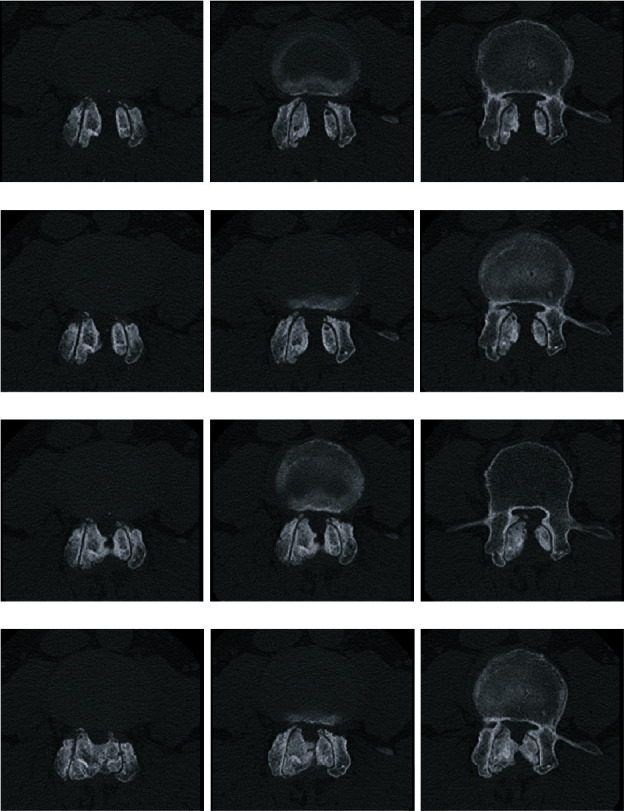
Computed tomography (CT) images after the second decompression surgery. (a) CT images 6 months after the second surgery. (b) CT images 1 year after the second surgery. (c) CT images 2 years after the second surgery showing bone regrowth mainly around the facet joints. (d) CT images 4.3 years after the second surgery showing the marked bone regrowth over the lamina.
